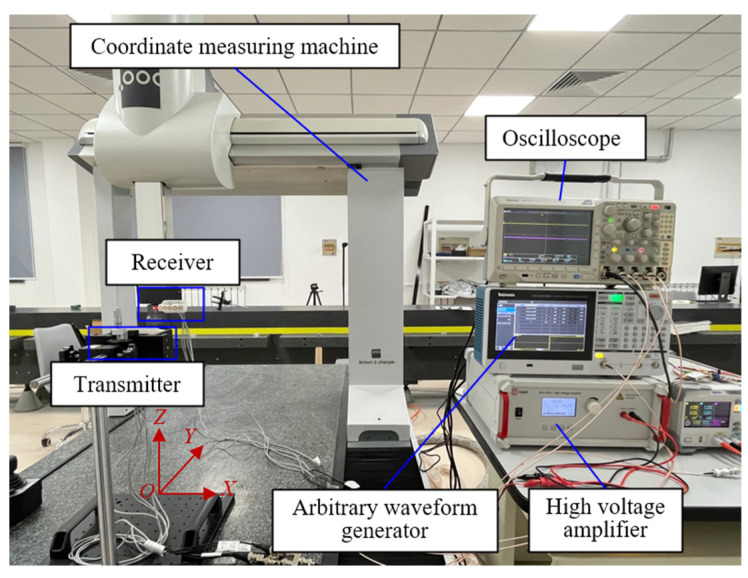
The experiment platform of ultrasonic static measurement.
